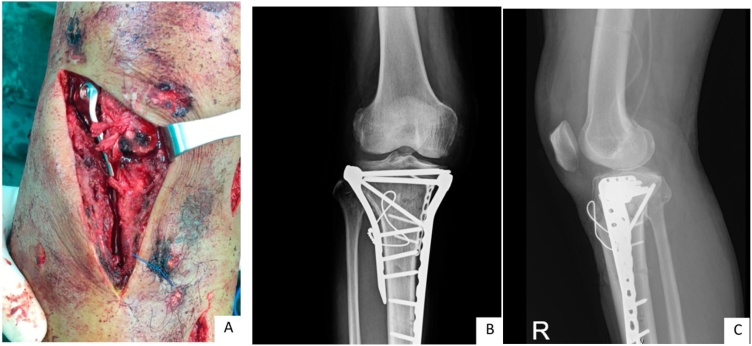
The clinical imaging (A) and radiographic imaging in (B) anteroposterior view, (C) lateral view of right knee.
